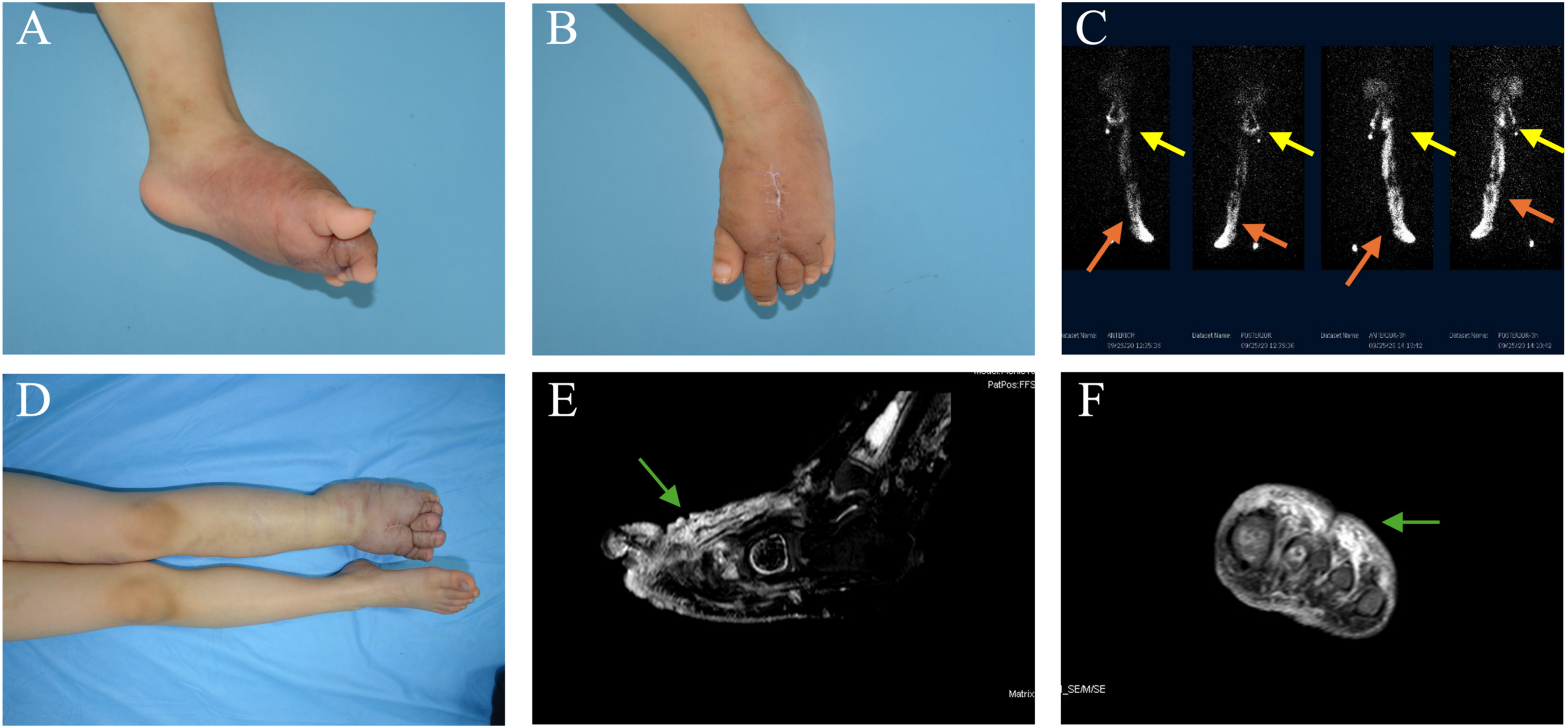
A female patient with KHE affecting the left foot presented with lymphedema. KHE onset occurred at 2 years of age, and limb swelling occurred 2 years later, at the age of 4. (A,B) Photographs taken at the time of referral depict swelling of the left foot, along with an old surgical scar on the dorsum of the foot. (C) Radionuclide lymphoscintigraphy revealed uptake in the bilateral inguinal and pelvic lymph nodes at 100 and 150 min after injection into the left foot (yellow arrows). Abnormal tracer accumulation and dermal reflux were observed in the left lower limb (marked by the concentrated white area, as shown by the orange arrows). (D) After 33 months of combined drug therapy and physical therapy, worsening swelling was observed in the left foot. (E,F) Sagittal and coronal T2-weighted images revealed high-intensity lesions in the dermis, subcutaneous tissue, and deep fascia of the left foot, accompanied by soft tissue swelling around the lesions (green arrows).
